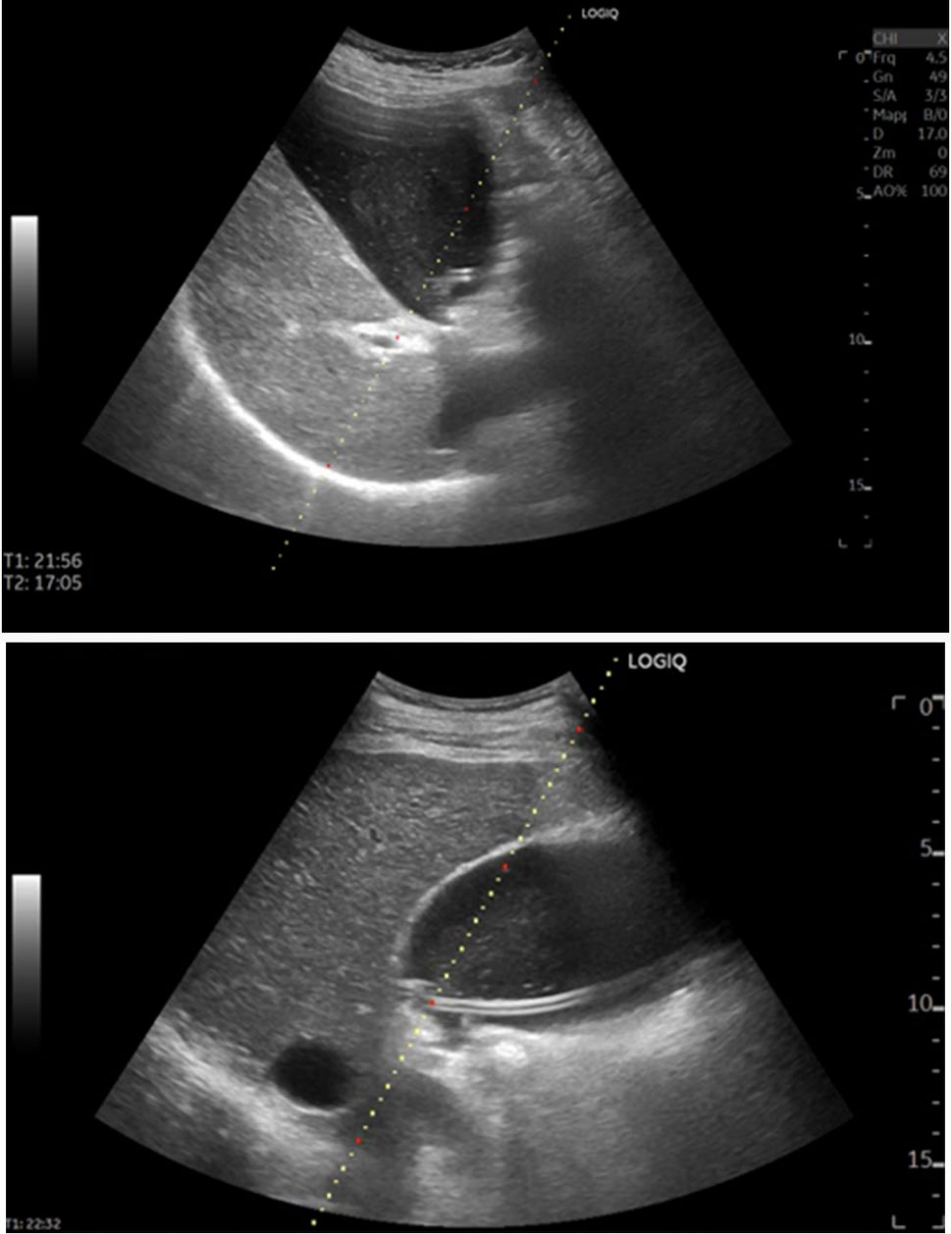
Screen right-upper-quadrant ultrasound with grayscale B-mode. An 8-Fr percutaneous pigtail drain is visible within the gallbladder (echogenic tubular structure with intraluminal course), consistent with transhepatic cholecystostomy; the pigtail tip is seen curled in the lumen. Coexisting gallstones produce echogenic foci with posterior acoustic shadowing
